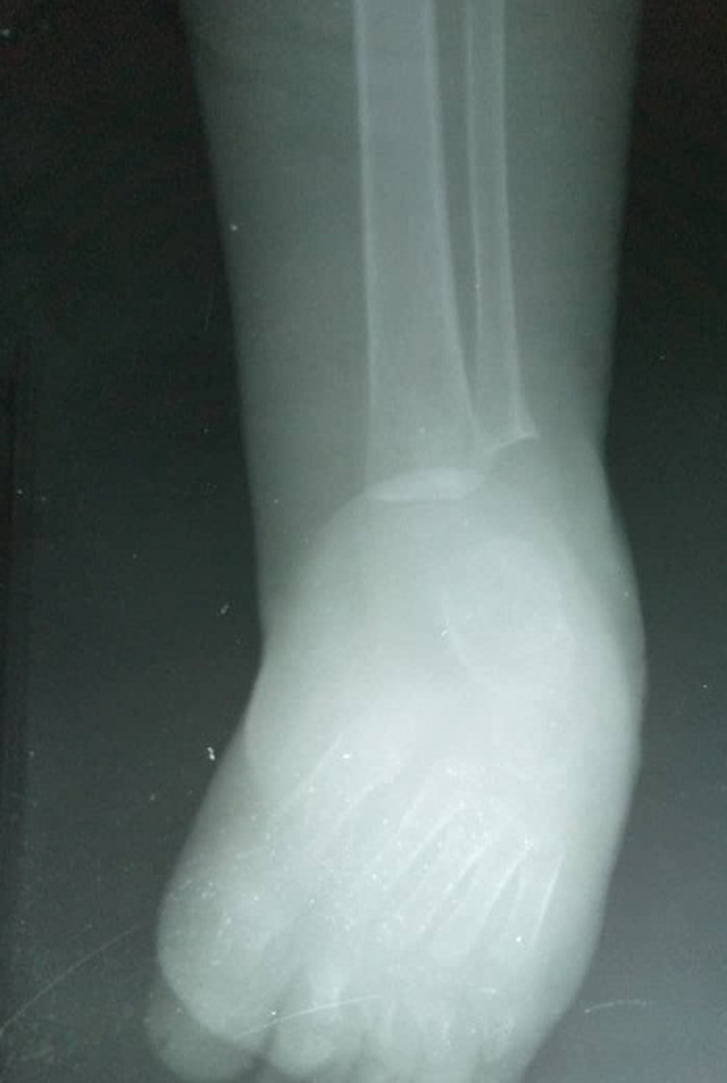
X-ray of RT distal part of the lower limb; note the lateral and medial malleolus of tibia and fibula respectively; the 3 cuneiforms, calcaneus, cuboid, navicular and talus
